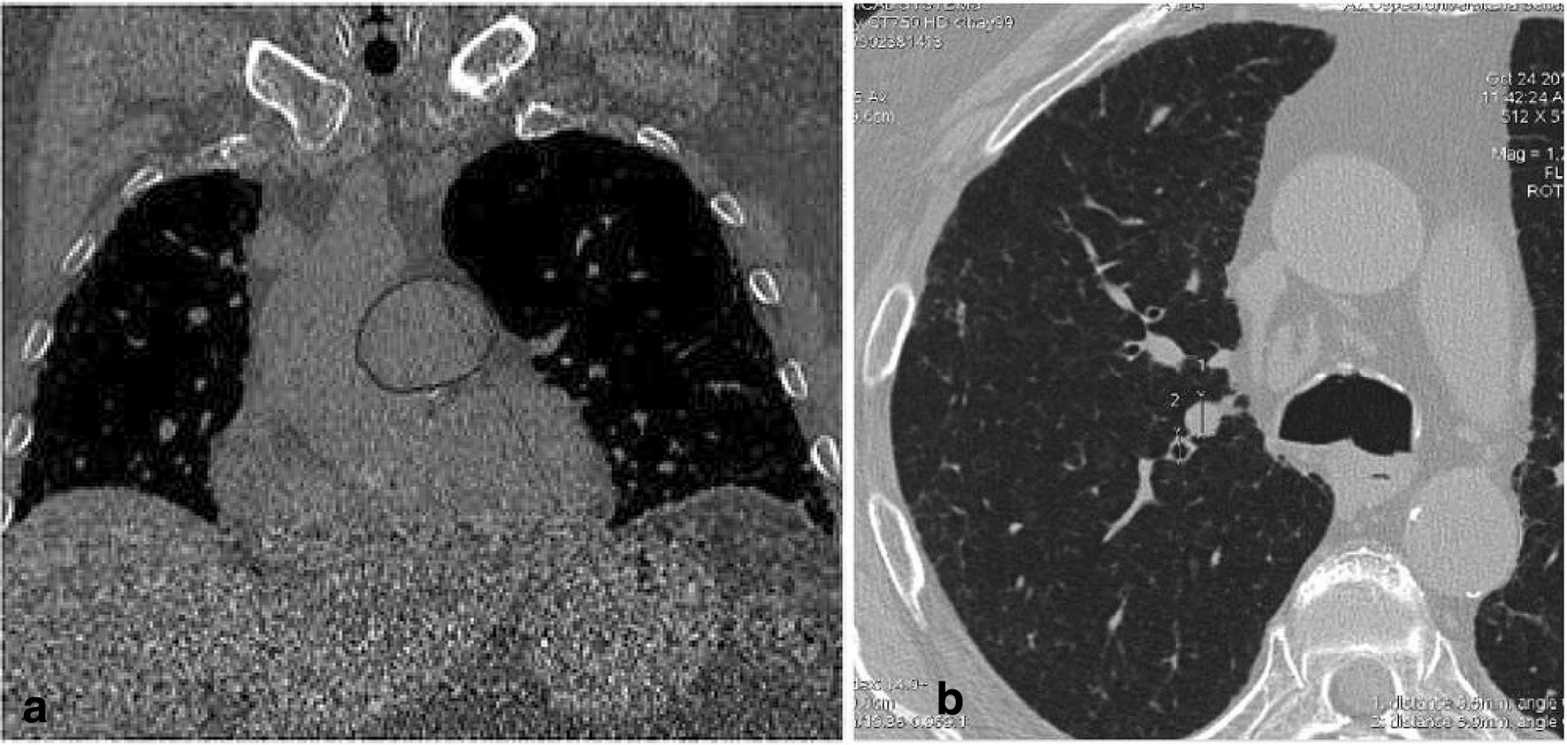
a The evaluated HRCT parameters: area of the *main pulmonary artery* (PA), before its bifurcation, on coronal reformation. b The widest short-axis diameters of the artery and bronchus of the apical segment of the right upper lobe
